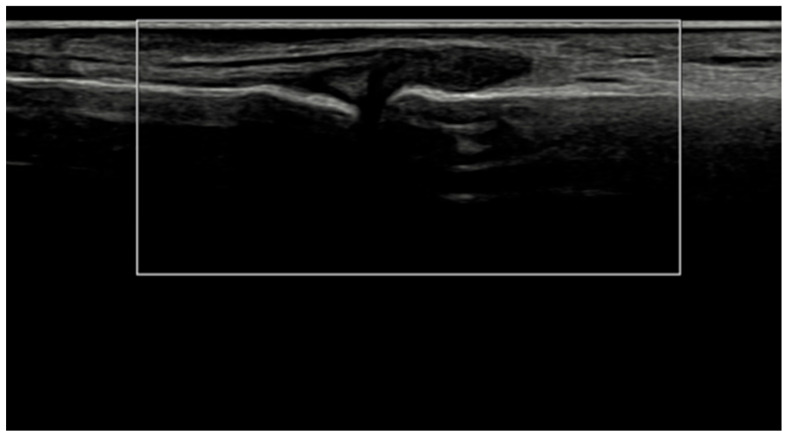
Longitudinal ultrasound image of the PIP II joint showing synovial hypertrophy (grayscale grade 2) without detectable intra-articular power Doppler signal (PD grade 0), corresponding to a combined EULAR–OMERACT synovitis grade = 2 (joint-level).
